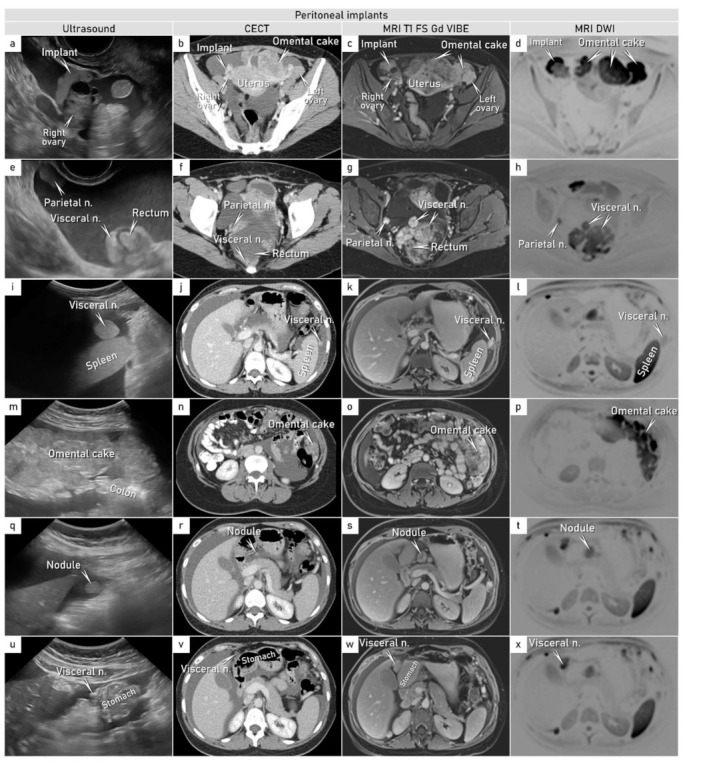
Peritoneal involvement (pelvis and abdomen). Ultrasound, contrast-enhanced computed tomography, and magnetic resonance with diffusion-weighted images demonstrating peritoneal metastases in transverse plane (unless stated otherwise): (a–d) Peritoneal visceral implant on the right non-infiltrated ovary. (e–h) Parietal isolated nodule on the right pelvic side wall and diffuse visceral peritoneal involvement on rectosigmoid. (i–l) Visceral focal nodule on the splenic surface. (m–p) Diffuse omental infiltration (omental cake). (q–x) Visceral nodules in the omental bursa and on the stomach (in sagittal plane on ultrasound). Abbreviations: n., nodule(s); MRI T1 FS Gd VIBE, T1-weighted magnetic resonance imaging after intravenous gadolinium; VIBE, volumetric interpolated breath-hold examination; CECT, contrast-enhanced computed tomography; DWI, diffusion weighted imaging. Supplementary Video S1.
